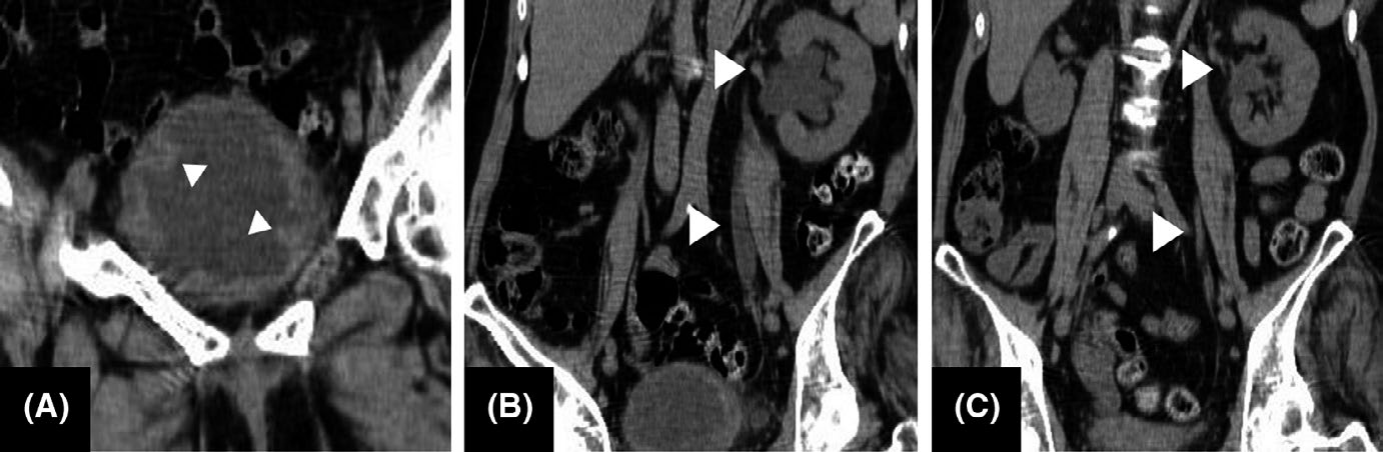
Abdominal CT images taken at admission showed trabeculated bladder (A) and dilation of left renal pelvis and ureter (B). CT image on day 15 showed reduction of dilated urinary tract (C)
